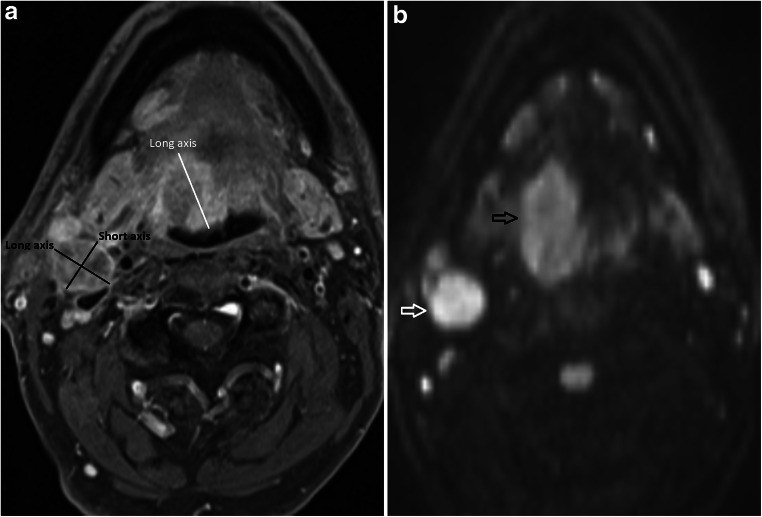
Measurable right base of tongue carcinoma and largest right level 2 lymph node to demonstrate dimensions evaluated. a Axial fat-saturated post-gadolinium T1w image demonstrates the long axial primary tumour measurement (white line), and both the long and short axial largest lymph node measurements (black lines) are depicted. There is necrotic/cystic change recorded in the largest lymph node. b DWI *b* = 800 axial image is used to aid the delineation of tumour. The highest DWI signal of the tumour (black open arrow) was recorded as 2 (isointense to cord) and that of the lymph node (white open arrow) was recorded as 3 (moderately hyperintense to cord)
